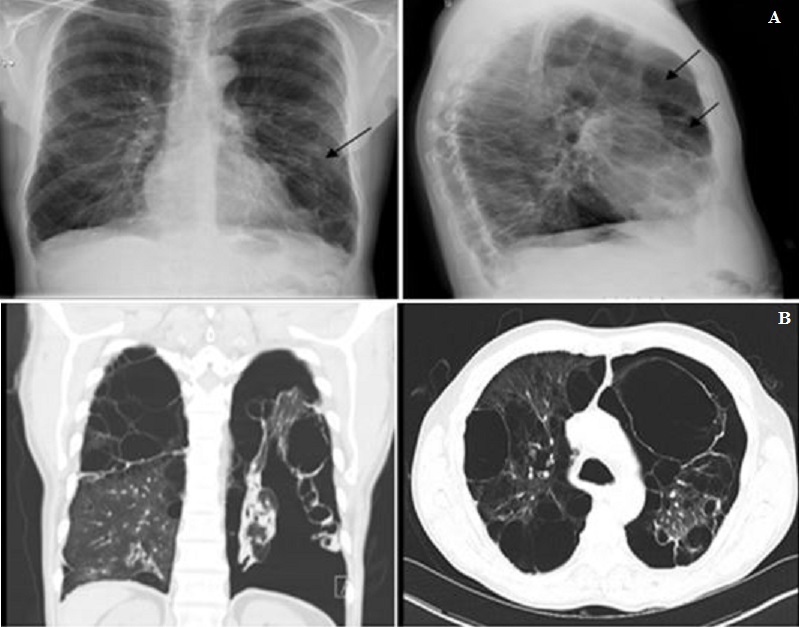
(A) PA and lateral views of the chest X-ray showing severe emphysematous changes; (B) axial and coronal views of the chest CT showing extensive bilateral bullous lung disease with collapse of surrounding parenchyma
